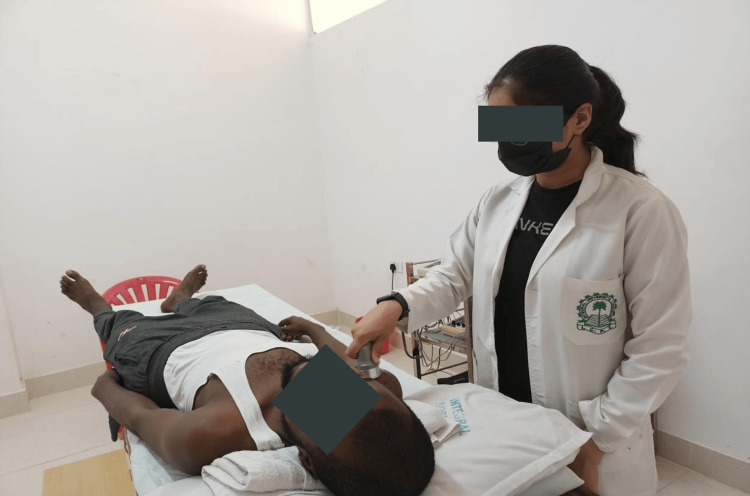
Application of ultrasound therapy at the glenohumeral joint The image is clicked by the author.
